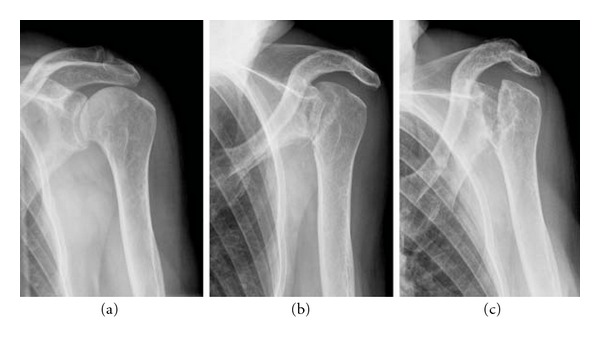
Plain true a.p. X-rays of the left shoulder in the same patient showing progressive osteolyses of the humeral head, the acromion, the lateral clavicle, and the glenoid in Gorham-Stout disease.
